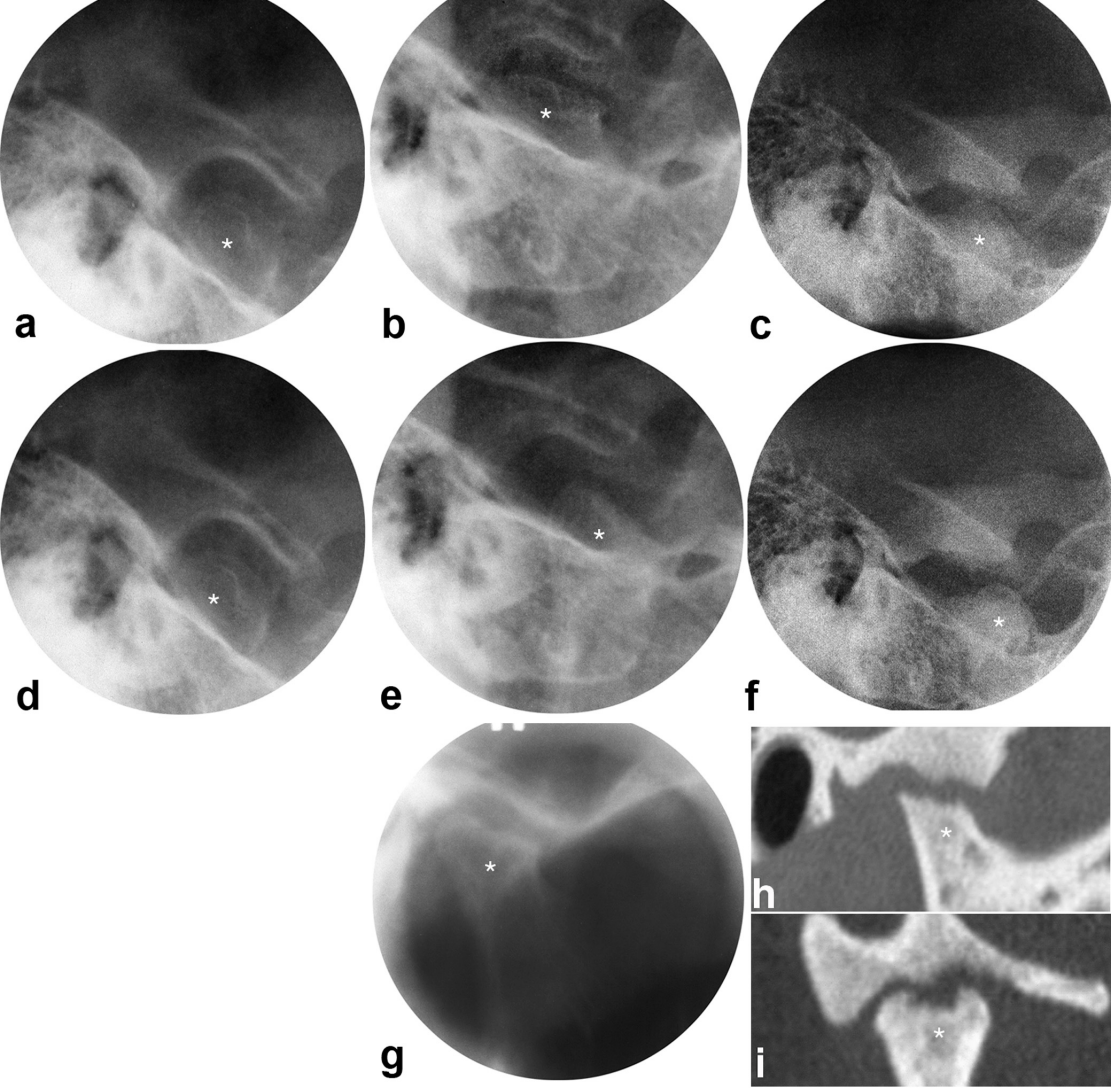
JIA patient with consistently progressing disease course in the TMJs. Transcranial radiographs at closed (a,b,c) and maximally opened mouth (d,e,f), including tomogram (g), and oblique sagittal (h) and oblique coronal (i) CT at closed mouth. One joint shown from 10 to 38 yrs with small bone abnormality and severely impaired condyle translation at baseline (a, d), moderate abnormality at 2 yrs follow-up (b,e,g) and extensive abnormality at final follow-up (c,f,h,i). * = mandibular condyle. Mandibular growth rotation shown in Fig. 2b.
